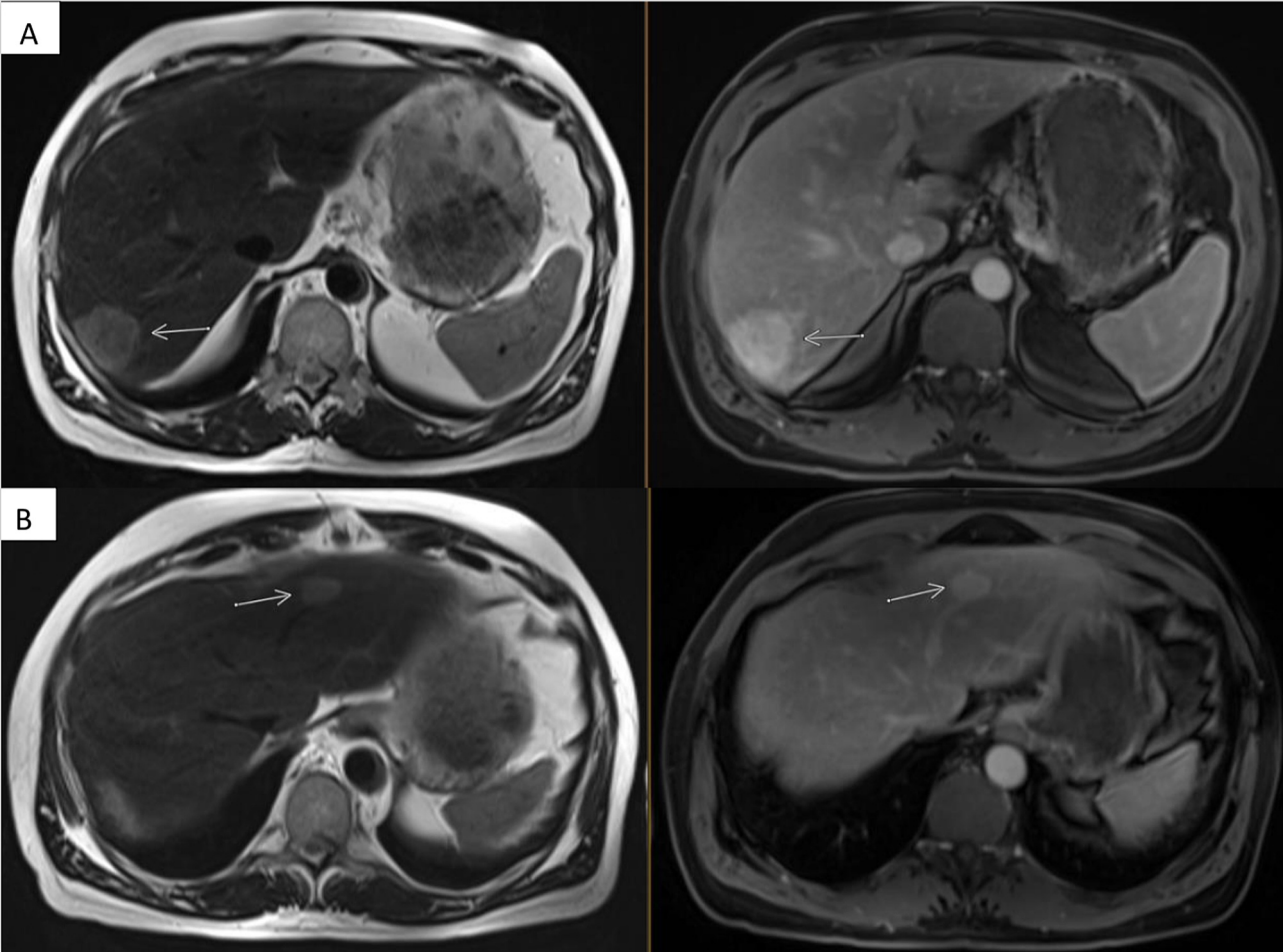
Interim MRI images while on adjuvant chemotherapy A: Axial T2 weighted image through the liver demonstrates increased T2 signal and conspicuity of the right hepatic lobe mass (arrow, left). Contrast-enhanced imaging was performed with an extracellular agent (gadobutrol) for this MRI. A three-minute post-contrast equilibrium phase image in the axial plane shows delayed enhancement of the mass (arrow, right). B: Axial T2 weighted image and three-minute post-contrast equilibrium phase showing similar T2 hyperintensity and delayed enhancement in the left hepatic lobe mass (arrows).
